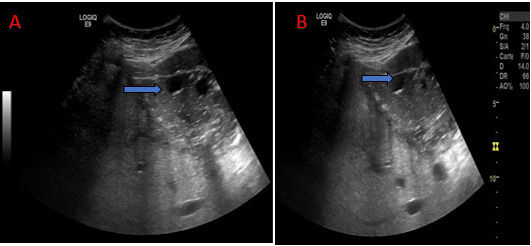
Abdominal ultrasound: Multi loculated cystic mass of the left lobe, stage III
